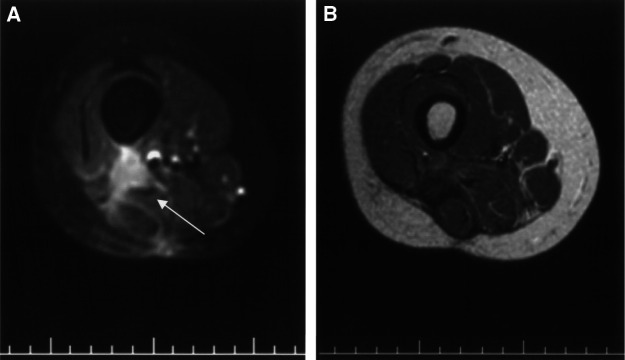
(A) Magnetic resonance (SE FS T1W post Gd-DTPA Image) showed a local relapse of right-thigh aggressive fibromatosis (arrow). (B) A partial response of disease (WHO criteria) was obtained by treating with ^90^Y-DOTATOC (SE T1W post Gd-DTPA magnetic resonance image).
